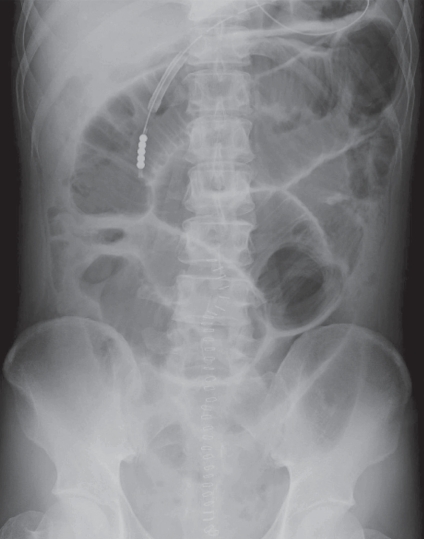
Abdominal plain radiograph of case 2 on admission showed multiple dilated gas-filled small-bowel loops, with no dilatation of the colon one day after undergoing surgical treatment.
